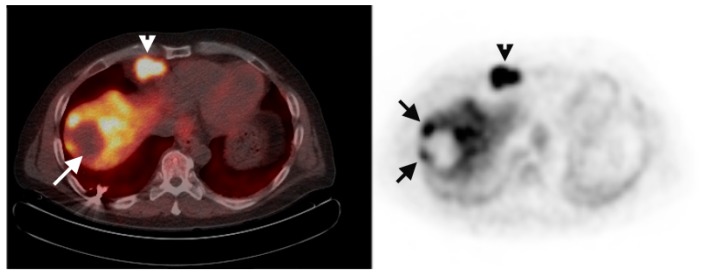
Recurrent HCC surrounding previous radiofrequency ablation site. PET/CT (left) shows absent FCh uptake (*white arrow*) in an area of the liver where HCC was previously treated by radiofrequency ablation. Corresponding PET (right) clearly shows multiple foci of increased FCh uptake surrounding the ablation site consistent with recurrent HCC (*arrows*). A pulmonary metastasis was also detected in this patient (*arrowheads*).
